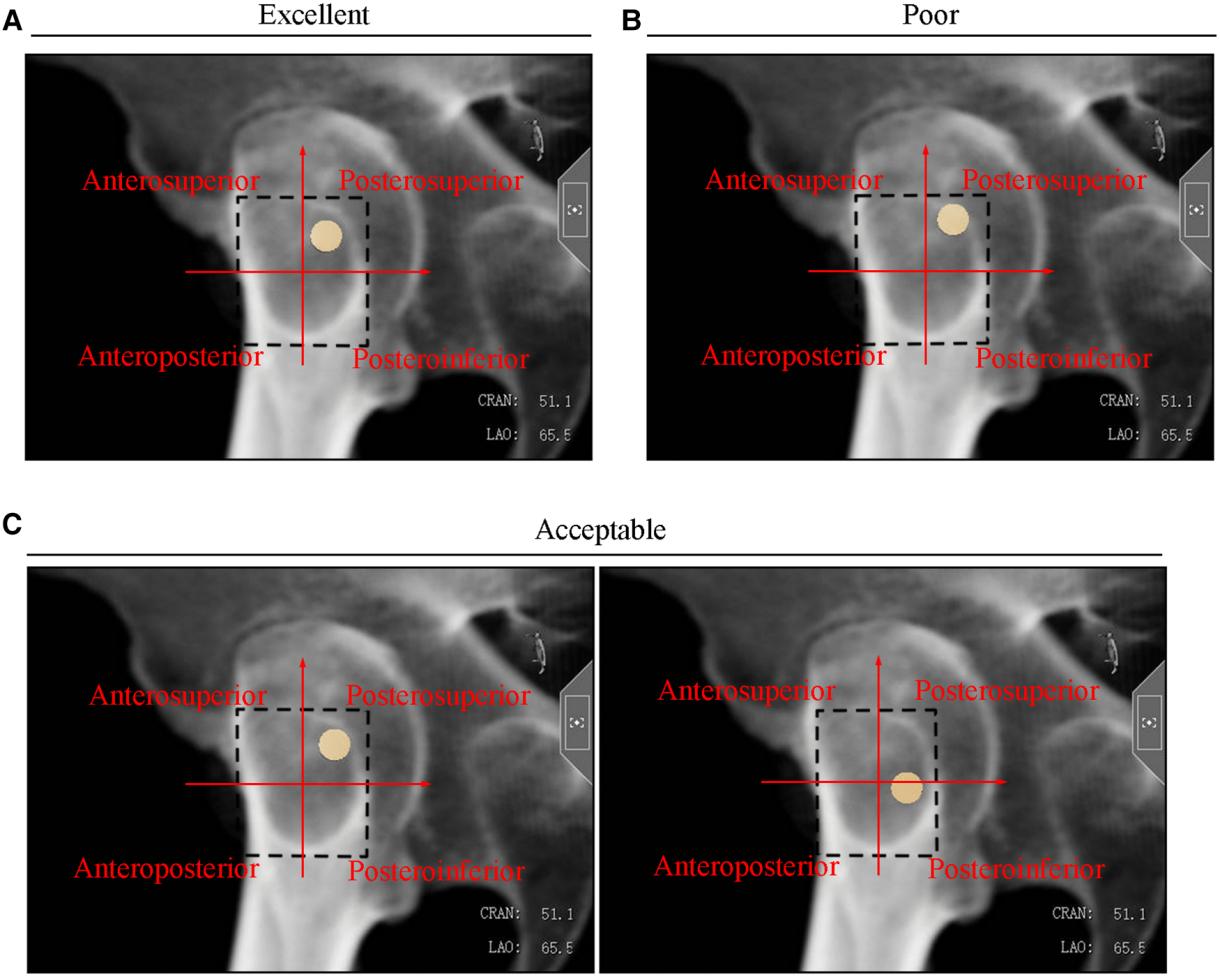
Typical (A) excellent, (B) poor, and (C) acceptable screw positions in the axial radiograph.
